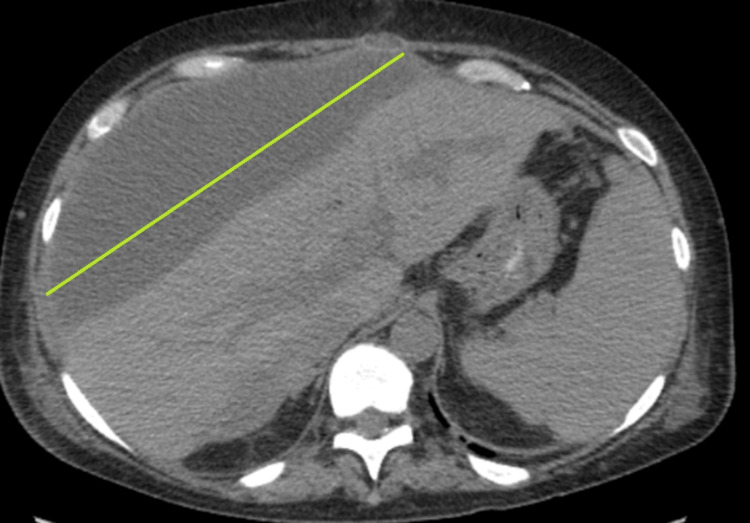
CT abdomen/pelvis showing a perihepatic collection measure of 25 cm (green line), indicating possible bile leak or biliary injury
